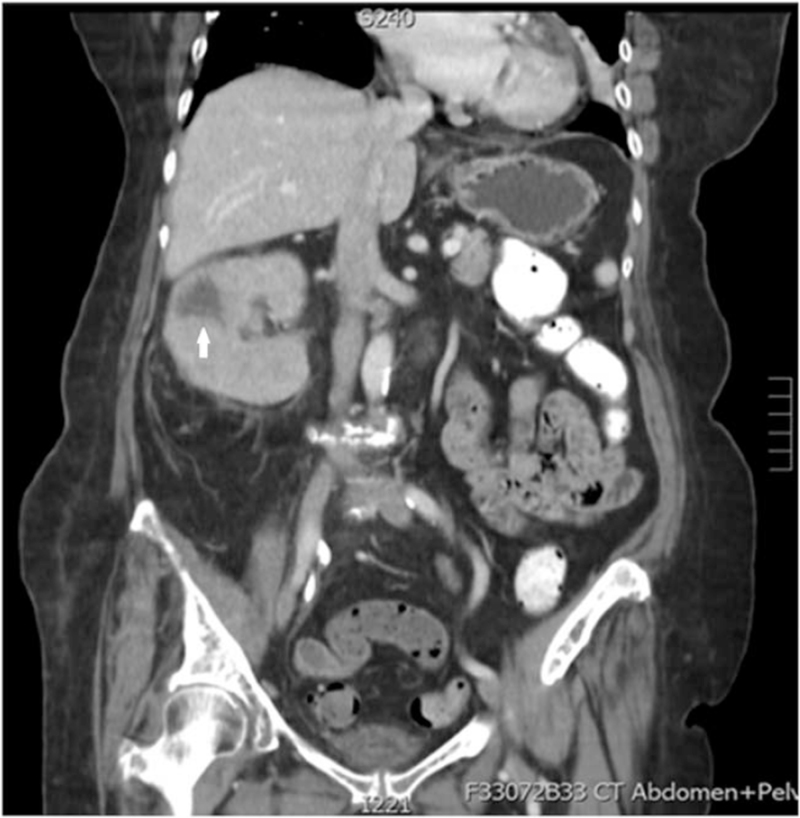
Computed tomography (CT) image. The enlarged right kidney (11.2 × 6.5 × 4.5 cm in size) with a low-density lesion (3.3 × 3.1 × 3.0 cm in size) at the right middle calyx, and a well-enhanced ureter were noted on the computed tomography (CT) scan. The white arrowhead indicates the tumor.
